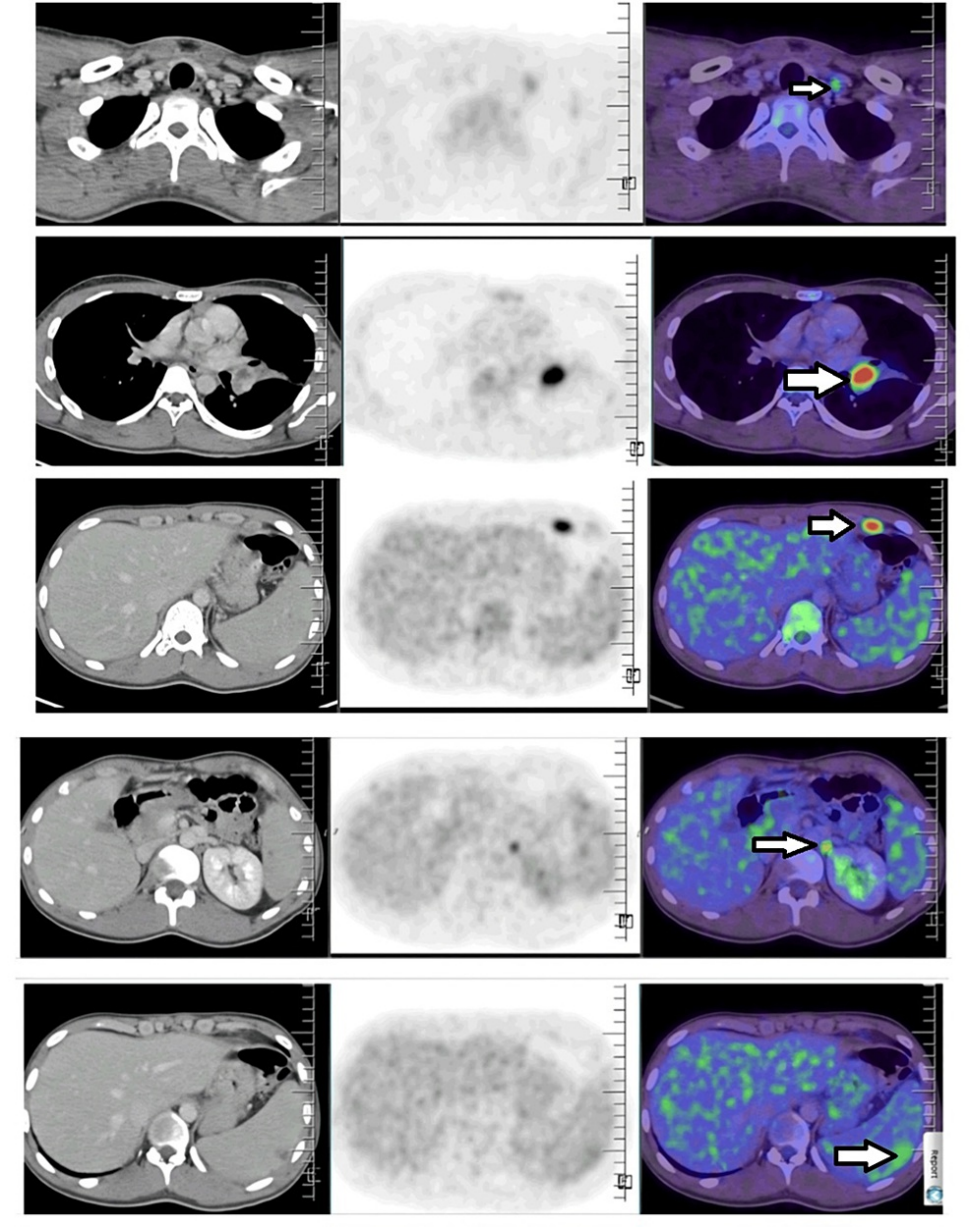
PET-CT scan showing FDG avid lymph nodes and splenic microabscess (arrows). FDG: fluorodeoxyglucose
